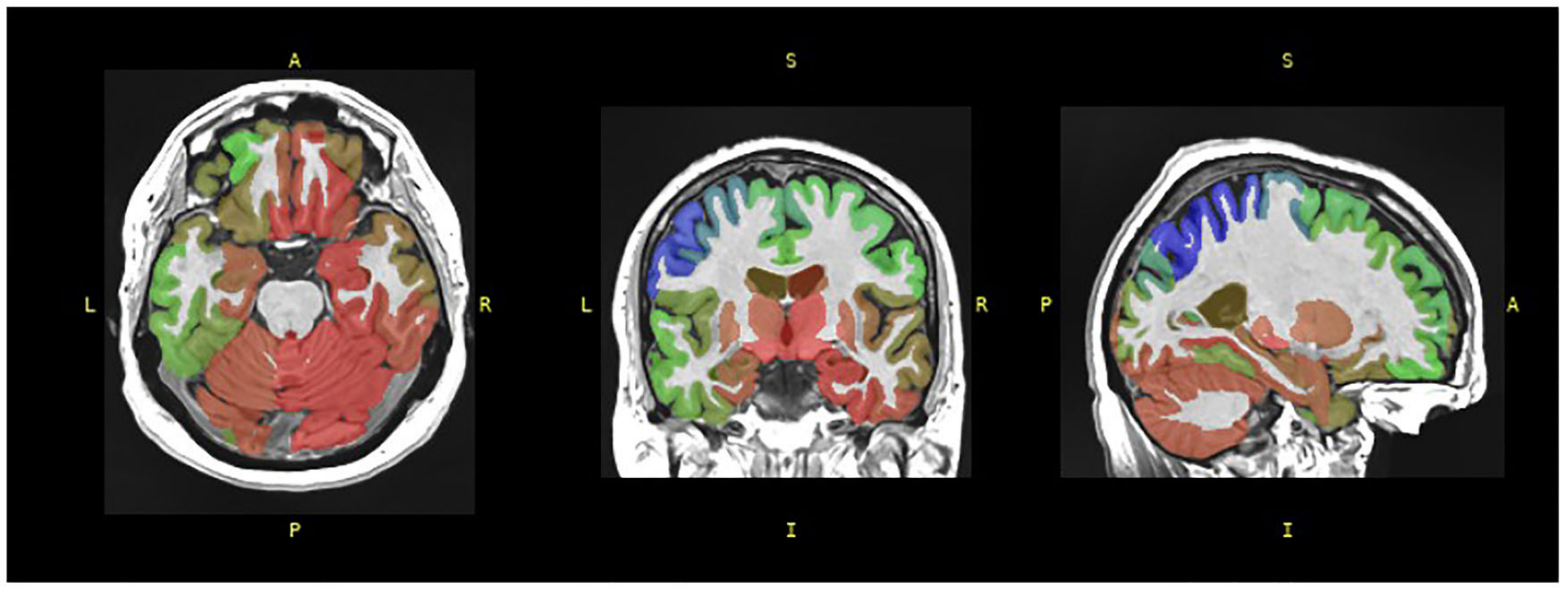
A structural analysis of T1‐weighted MRI was conducted using the volBrain platform to estimate (BSA. The estimated age range is expressed in years, with the chronological age of the PD patient recorded as 63 years.
